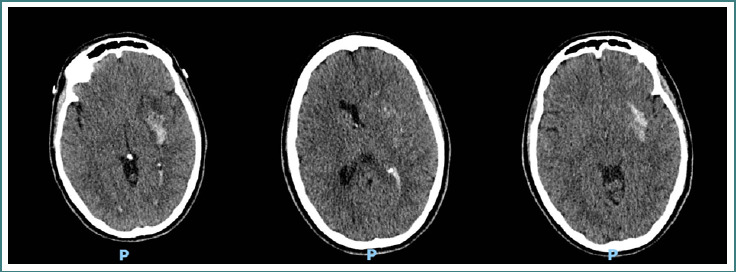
CT scan after extubation on day 5 with hemorrhagic transformation
